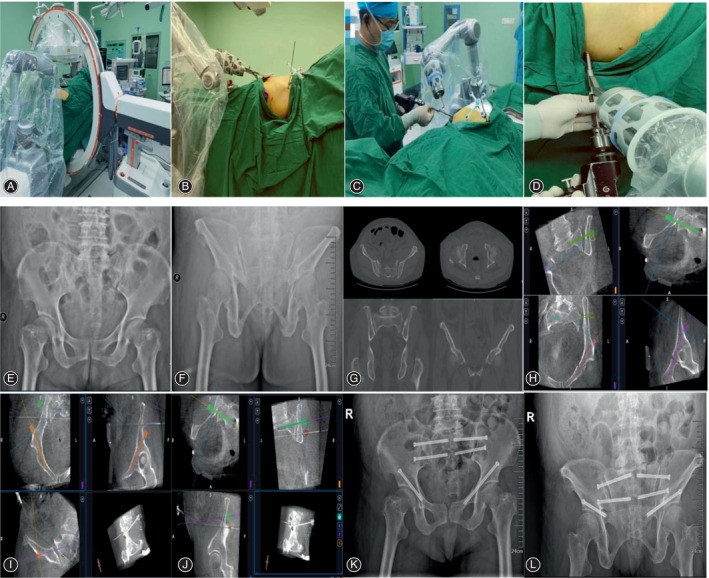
A 73‐year‐old woman with a fragility fracture of the pelvis (FFP IVa type). The surgical procedure of TiRobot‐assisted channel screw fixation. (A,B) C‐arm was used to capture intraoperative three‐dimensional (3D) images. (C,D) The surgeon placed the screws according to the 3D guide of the Tianji robotic arm, and the screw diameter was 6.5 mm to assist indirectly the fracture reduction. (E,F) Preoperative anteroposterior (AP) and outlet radiographs of the pelvis showed bilateral sacroiliac joint separation and bilateral acetabular anterior column fracture. (G) Horizontal and coronal CT of the pelvis: bilateral sacroiliac joint separation, left sacral fracture, bilateral sacroiliac joint “open‐book” injury, and bilateral acetabular anterior column fracture. (H–J) On the host interface of the “Tianji” orthopedic robot, screw placement for the sacroiliac joint was planned on both sides (S1/S2), and screw placement for the anteroposteric column was planned on both sides. Screw positions and lengths could be detected on 3D multi‐plane and 3D fluoroscopic images. (K,L) Postoperatively, anteroposterior (AP) and outlet pelvic radiographs showed good fracture reduction and internal fixation. Bilateral sacroiliac joint screws and bilateral anterograde anterior column screws were in good positions and did not invade the peri‐channel cortical bone.
